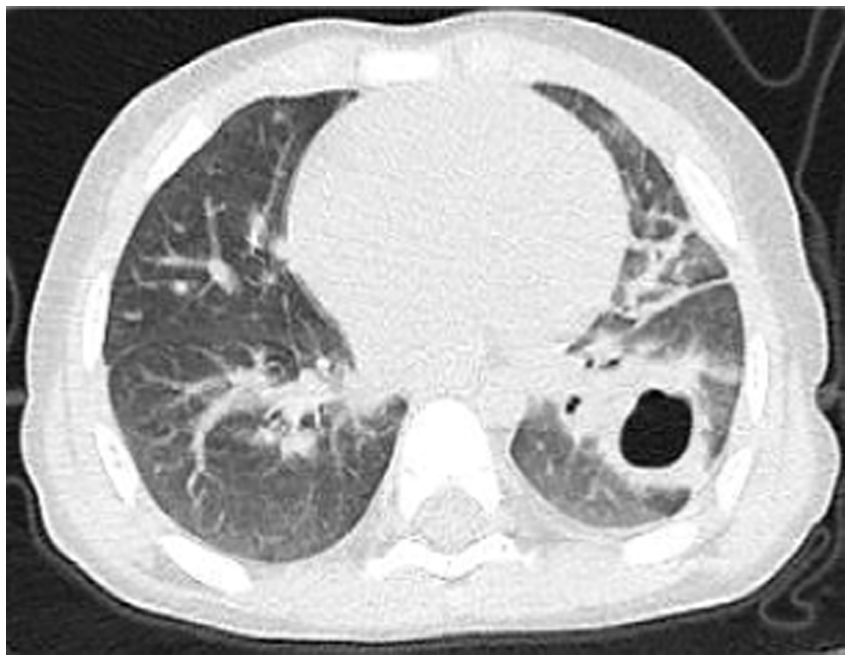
Chest CT scan on the 67th day of the illness.
